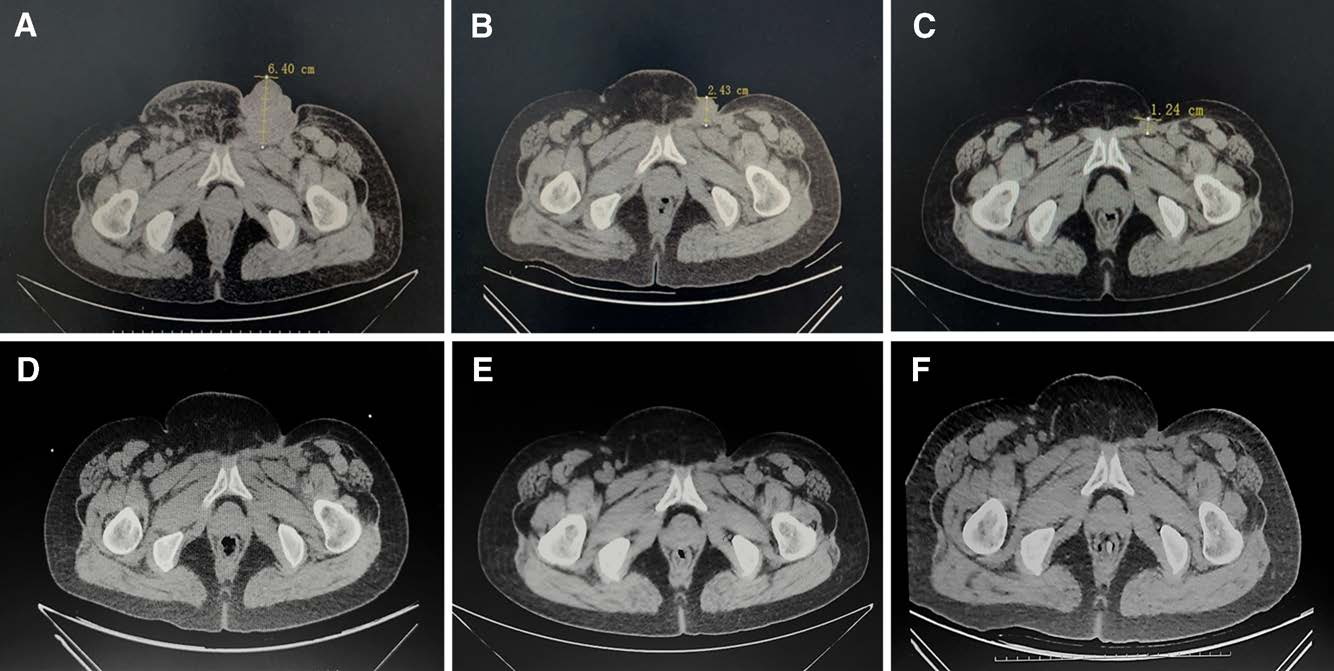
The pelvic CT images of the patient at different stages. (A) A pelvic CT scan at baseline showed a lobulated mass in the left inguinal region. (B) A pelvic CT scan (in May 2020) revealed the mass in the left inguinal region was significantly reduced after 2 cycles of immunotherapy, and the therapeutic effect was evaluated as PR. (C) A pelvic CT scan (in June 2020) demonstrated the mass in the left inguinal region had shrunk further after 4 cycles of immunotherapy, and the efficacy was evaluated as PR. (D) A pelvic CT scan (in December 2020) indicated no mass was in the left inguinal region. (E) A pelvic CT scan (in April 2021) indicated no mass was in the left inguinal region, and the disease was stable. (F) A pelvic CT scan (in August 2021) indicated the disease of the patient remained stable. CT = computed tomography, PR = partial response.
